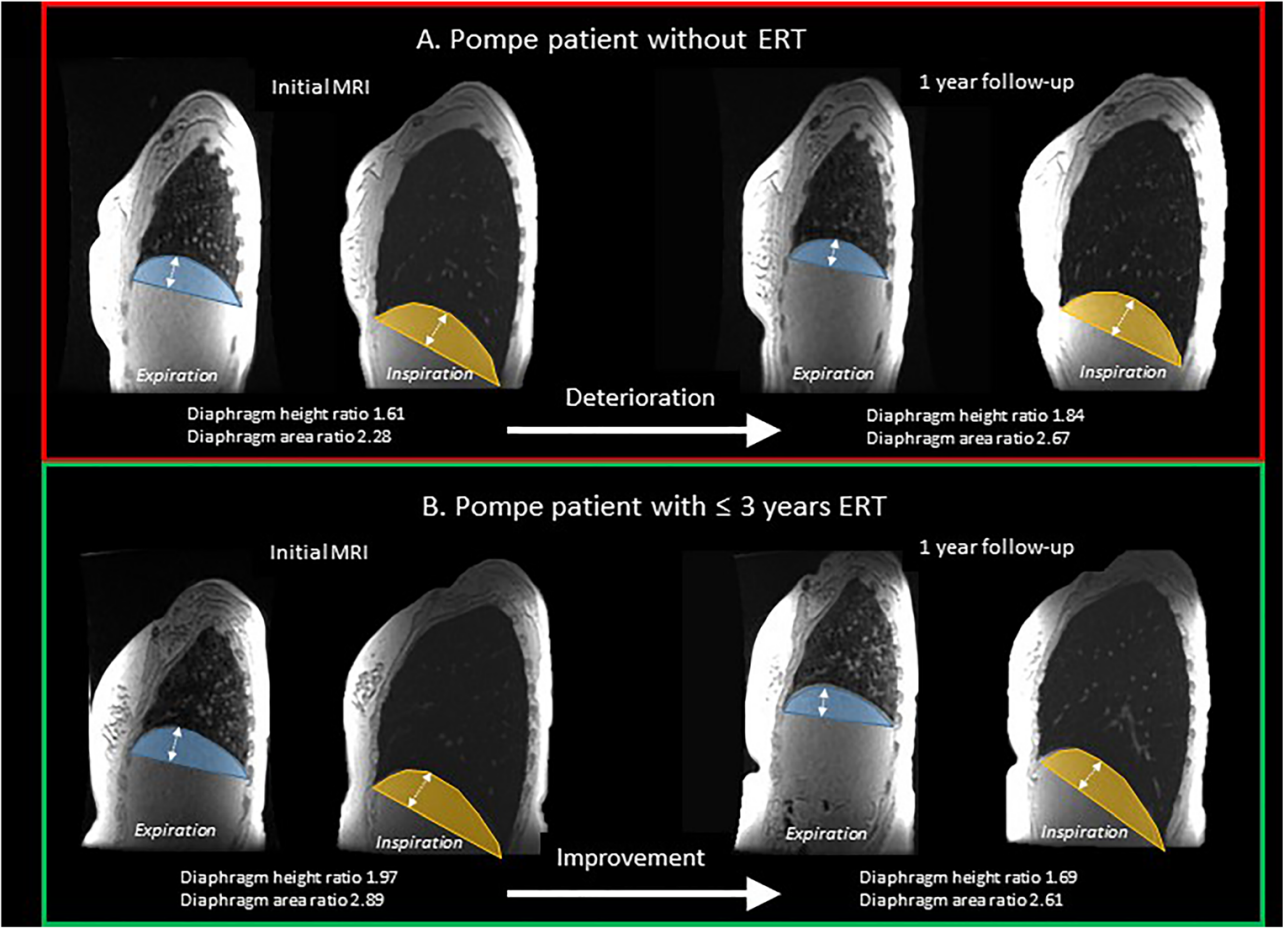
The diaphragm height is shown in white arrows. The diaphragm area is shown in blue (expiration) or yellow (inspiration). Ratios are outcomes at inspiration divided by outcomes at expiration. ERT, enzyme replacement therapy. Patient A is a female Pompe patient of 17 years old, not treated with ERT because she is asymptomatic and stable over time. At the initial MRI, both diaphragm height ratio and diaphragm ratio are increasing during inspiration (ratio > 1), indicating an insufficient diaphragmatic contraction. After 1-year follow-up, the ratios are increasing, corresponding to a deterioration of diaphragmatic curvature. At the initial measurement, forced vital capacity (FVC) upright was 93% predicted and FVC supine 95%, at follow-up FVC upright was 94% predicted and FVC supine 89%. Patient B is a female Pompe patient of 25 years old and 2.5 years treated with ERT at the initial MRI. FVC upright was 119% predicted and FVC supine 115%. The diaphragmatic curvature is increasing during inspiration (diaphragm height ratio and diaphragm ratio > 1), indicating an insufficient diaphragmatic contraction. After 1-year follow-up, FVC upright was 115% predicted and FVC supine 113%, but the diaphragmatic height and area ratios are decreased, corresponding to an improved diaphragmatic curvature
